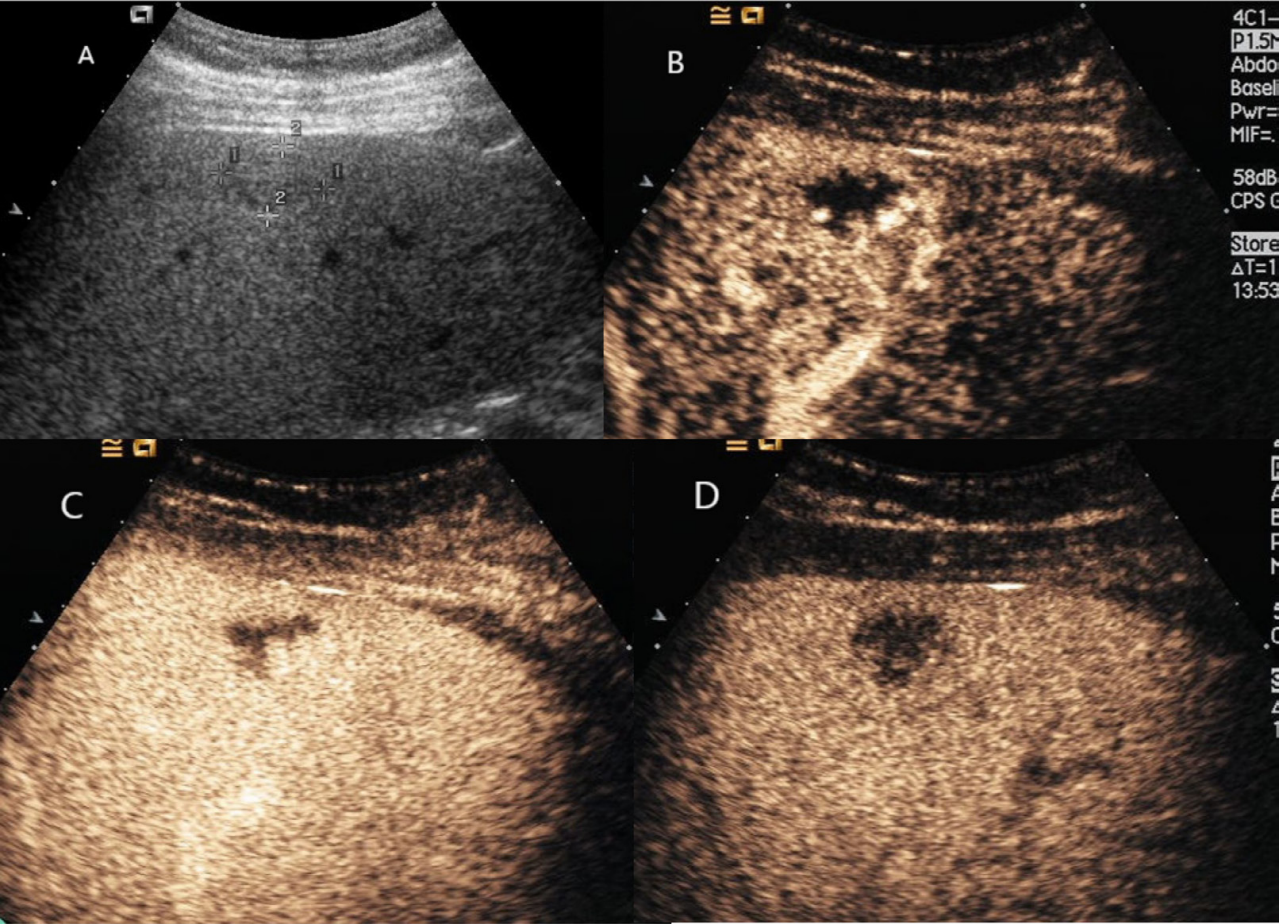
Hepatic hemangioma with washout in the delayed phase (A) Ultrasound revealed an isoechoic mass under right liver capsular. (B) and (C) CEUS showed peripheral nodular enhancement with centripedal filling. (D) CEUS showed hypoechoic change in the delayed phase.
